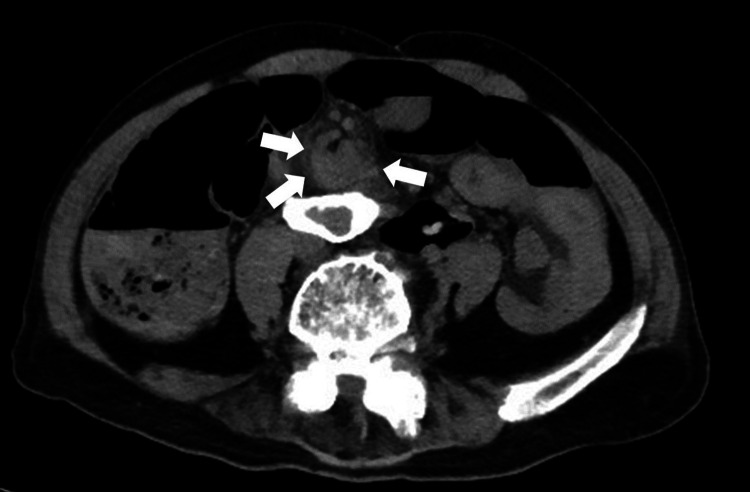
Recurrent sigmoid volvulus—axial non-contrast CT. Axial non-contrast CT demonstrates a mesenteric “whirl sign” centered on the sigmoid mesentery (white arrows) with marked upstream colonic dilatation, compatible with sigmoid volvulus.
